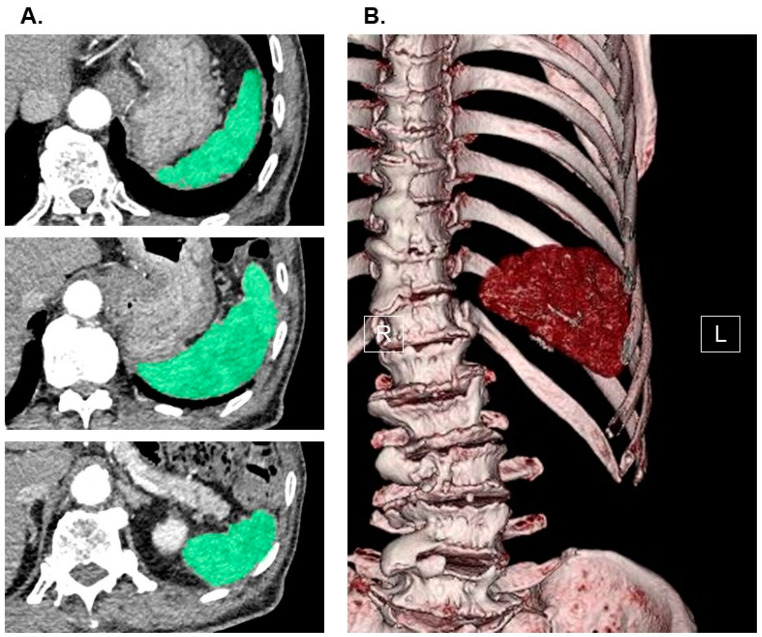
Measurement of splenic volume using abdominal computed tomography imaging. Spleen (green) in horizontal section was traced by the software in each slice (A). Three-dimensional image of spleen was displayed (B). SYNAPSE VINCENT software, version 2.0003, developed by Fujifilm in Tokyo, Japan, was used to measure splenic volume. Using the software’s preset algorithm for extracting elliptical tissue, the software automatically recognized the spleen when the long axis of the spleen was specified from a multi-slice computed tomography scan cross-sectional image (spleen was traced and colored in green in each slice). All the recognized splenic images were constructed in three dimensions and output as an image, and its splenic volume was automatically calculated. In cases where necessary, manual adjustments were made to the trace before arriving at the final measurements. For the independent variable, we indexed the value of splenic volume. R, right; L, left.
